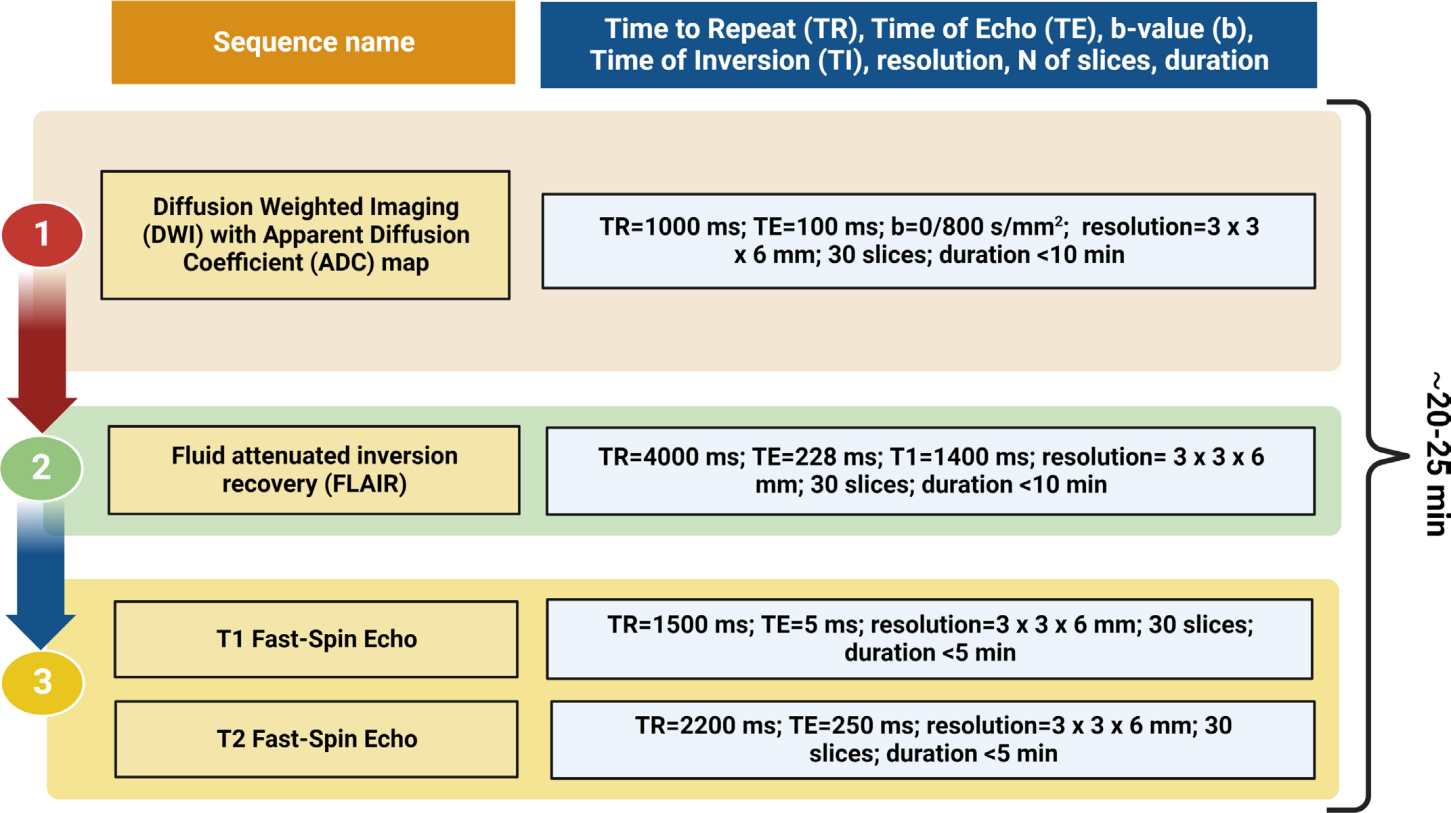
Prespecified low-field MRI minimum acquisition time pulse sequences protocol. DWI with ADC maps (1) will be acquired first to detect early stroke changes. Subsequently, FLAIR (2), T1 and T2 Fast-Spin Echo (3) sequences will be performed only when needed, according to clinical information (suspected stroke subtypes—eg, large vessel occlusion, potential treatment eligibility). The protocol is set up to have a total duration of ~20–25 min.
